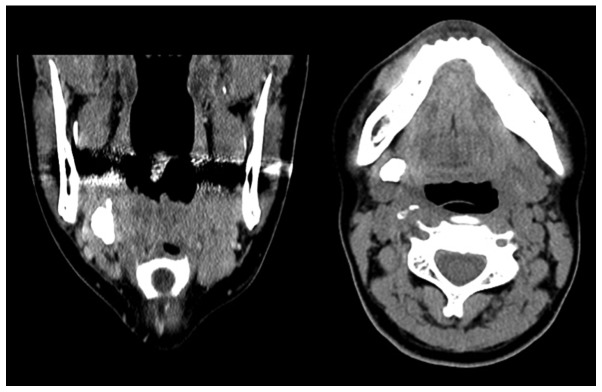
Preoperative contrast-enhanced computed tomography showing the salivary stone on the right submandibular gland.
